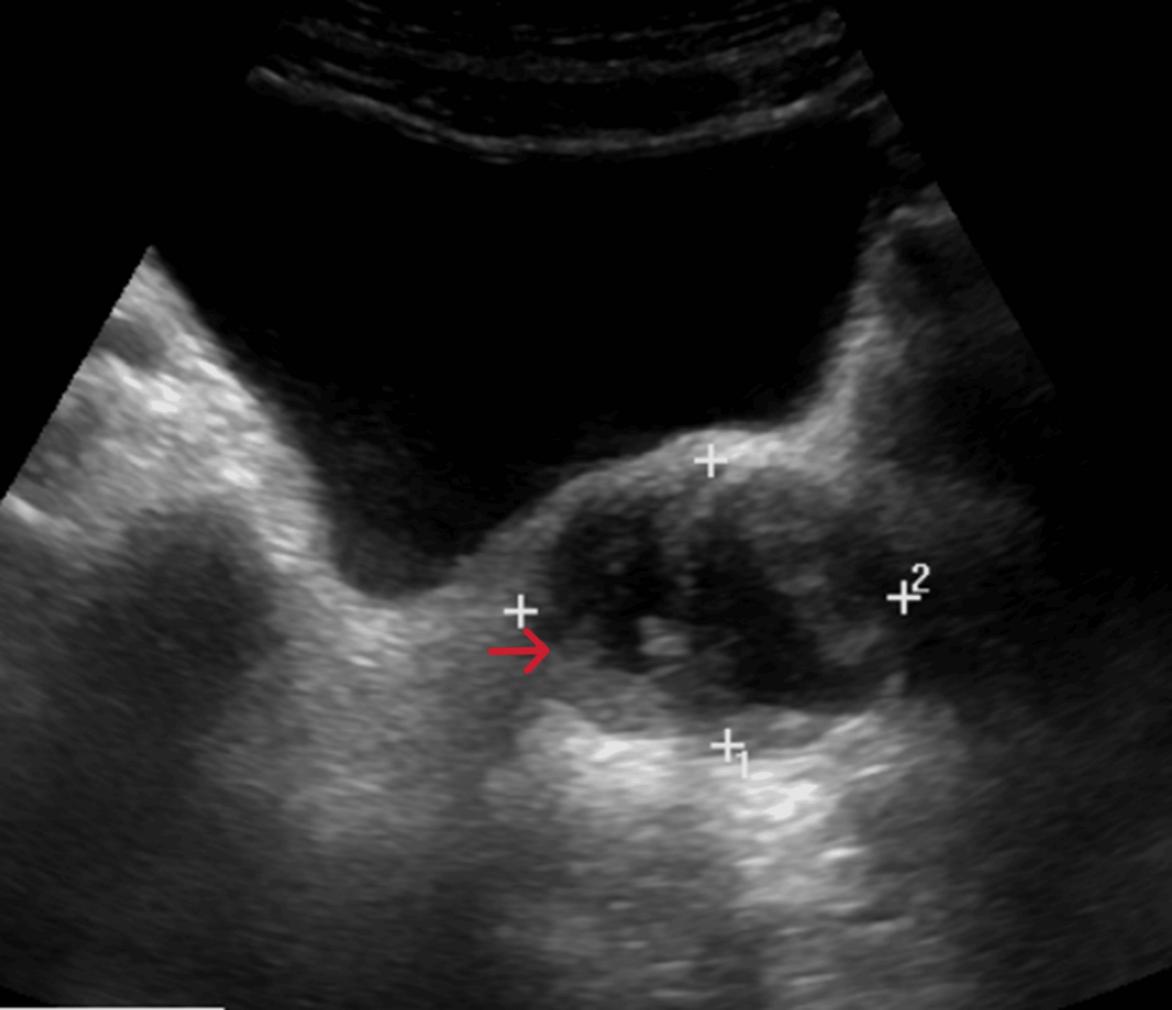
Ultrasound of the abdomen showing an enlarged prostate with a heterogeneous collection
